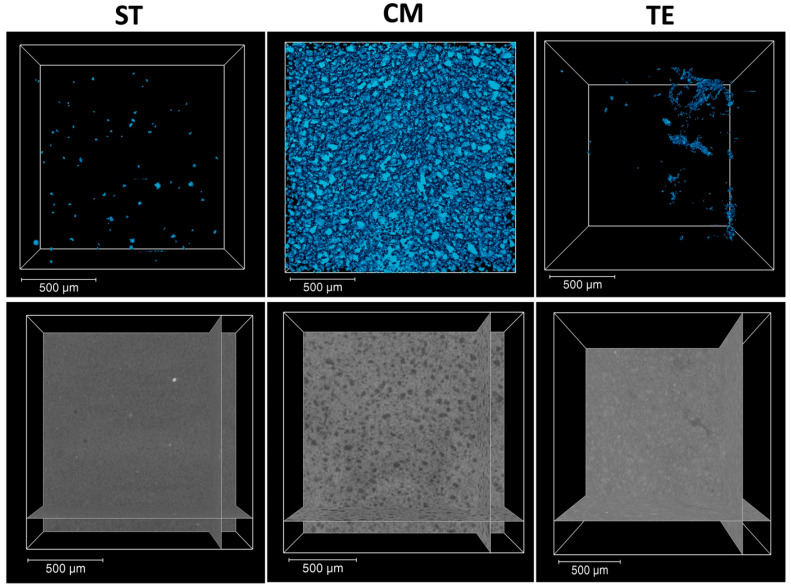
Volume rendering of segmented pores (blue color) with a scale bar of 500 µm, obtained by X-ray tomography analysis in ST (Stela Automix), CM (Clearfil Majesty ES-2), and TE (Tetric EvoCeram).
